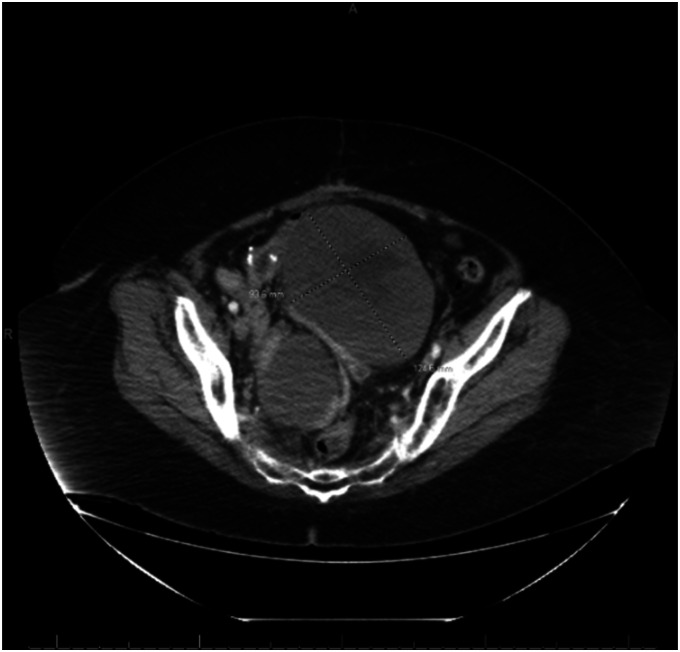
Two new pelvic masses, 12.5 cm and 9 cm, seen on computed tomography scan in 2023.
